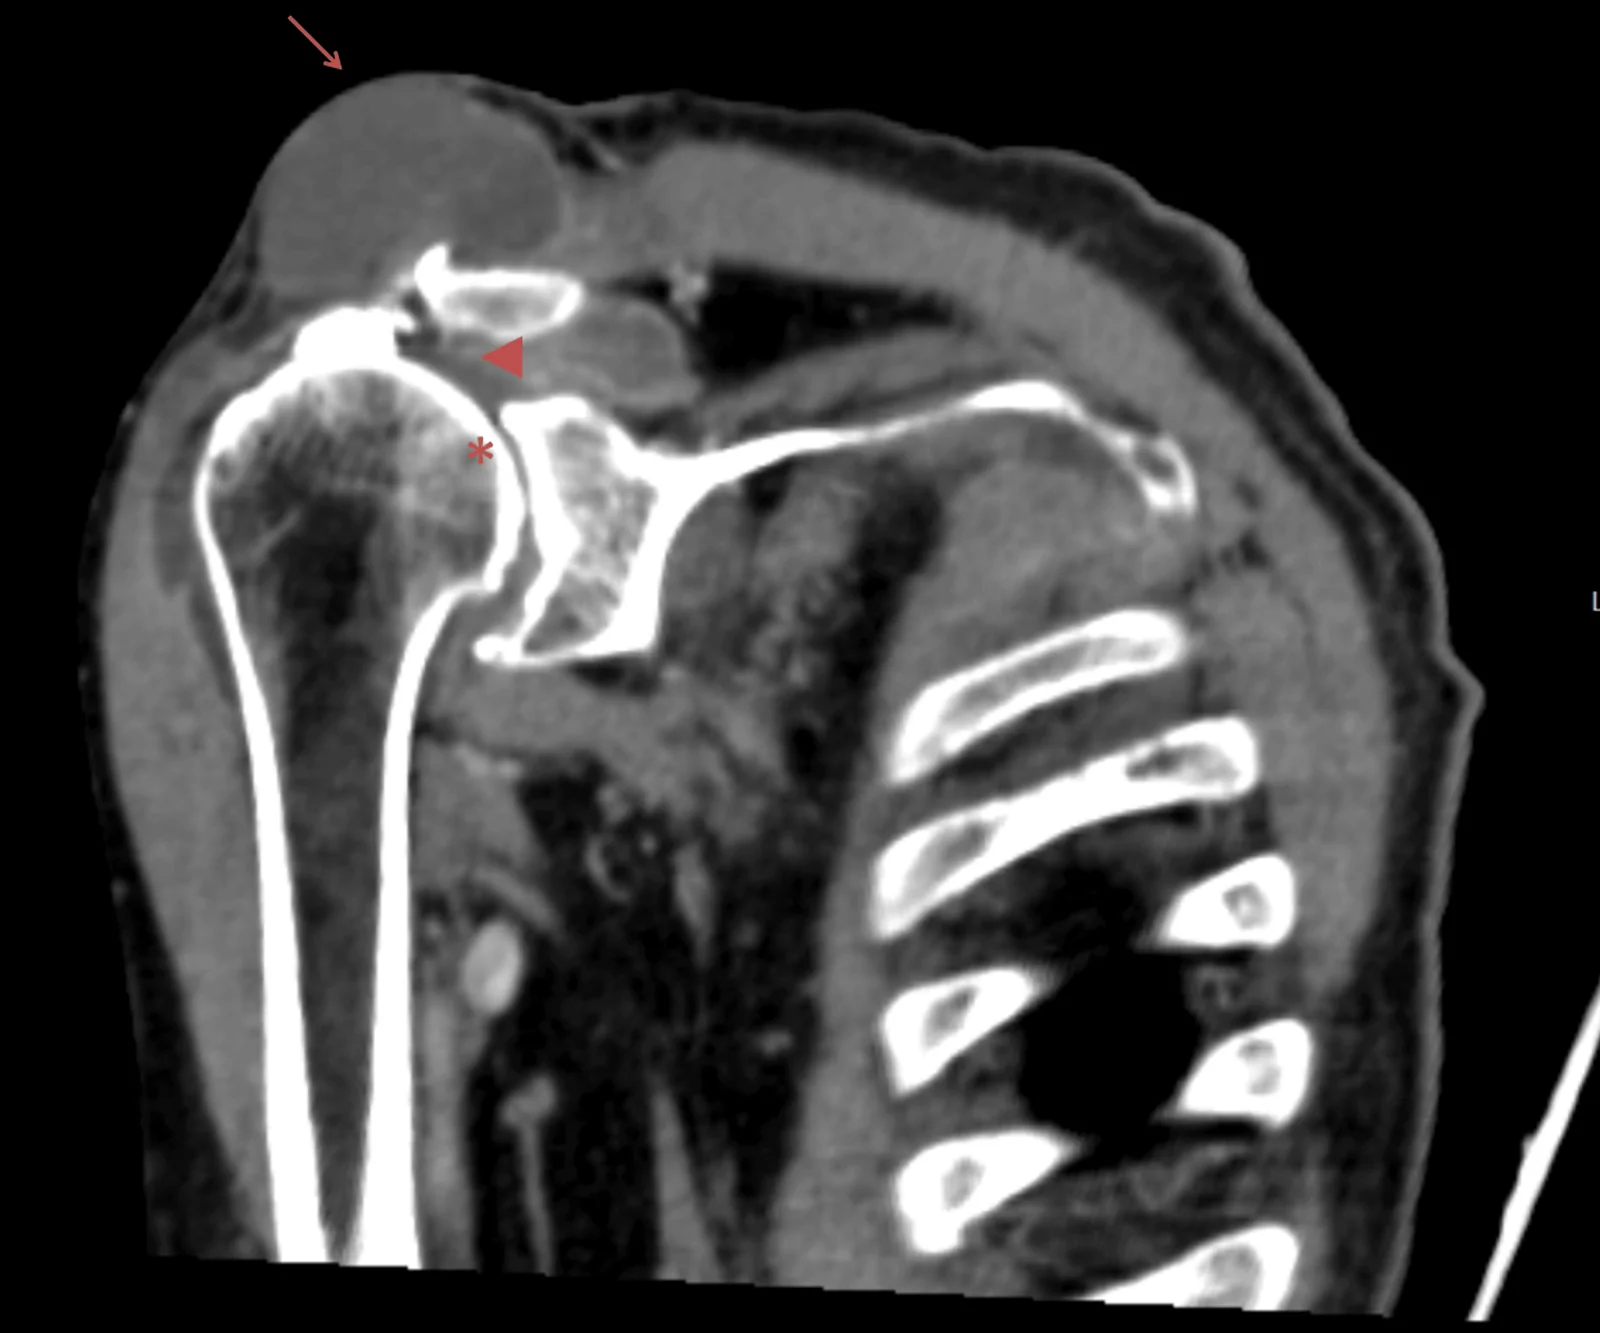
Coronal CT image of the right shoulder demonstrating an ACJ cyst (arrow), GHJ degenerative changes (asterisk), and a suspected superior fluid tract (arrowhead), compatible with GS. ACJ, acromioclavicular joint; GS, Geyser Sign; GHJ, glenohumeral joint
